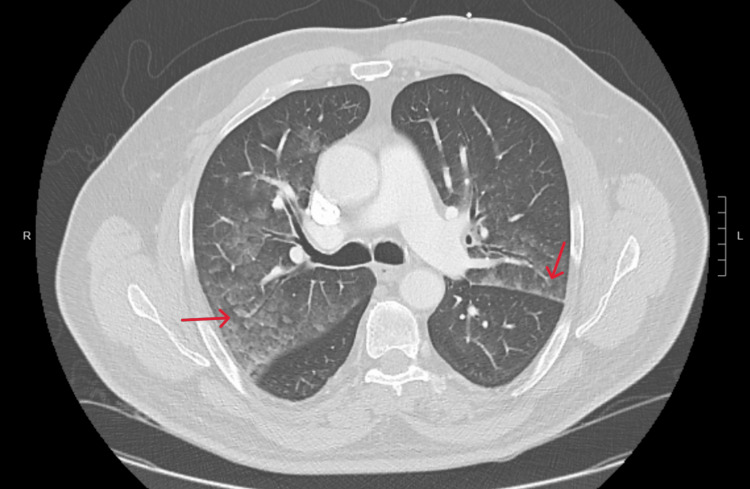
CT (axial plane) shows pulmonary edema. Red arrows indicate bilateral hazy increased attenuation signifying pulmonary edema. CT: computed tomography
